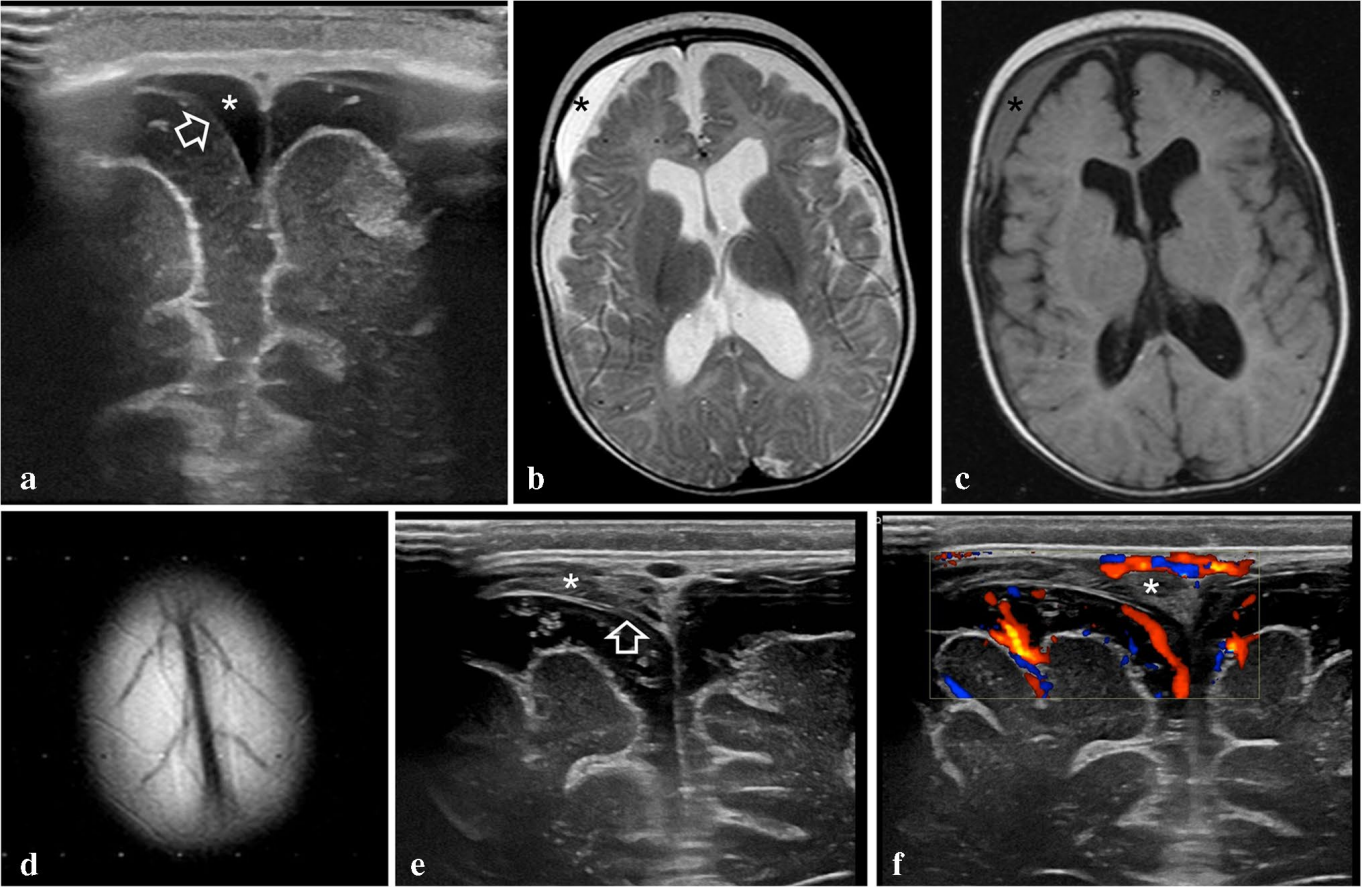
A 3-month-old ex-premature boy with progressive macrocrania and normal development. On ultrasound (US), there was an incidentally discovered subdural collection, confirmed by magnetic resonance imaging (MRI). Fundoscopy, skeletal surveys, social and family history, and clinical follow-up were unremarkable. The diagnosis was subdural collection in the setting of benign enlargement of the subarachnoid spaces. a Coronal US with a high-frequency linear transducer shows enlargement of subarachnoid spaces bilaterally and an anechoic subdural collection (*) displacing the arachnoid dura (*arrow*) on the right. A small linear membrane was also seen on the left. b MRI 2 weeks later. Axial T2-weighted image confirms increased craniocortical width and the presence of a hyperintense subdural collection (*). c MRI, axial FLAIR image, same level as in b. The subdural collection (*) is more conspicuous and restricts the adjacent subarachnoid space. d Axial T2-weighted image at thew convexity shows normal bridging veins. e Repeat ultrasound scan on the same day shows increased echogenicity of the subdural collection (*) while the arachnoid membrane is still visible (*arrow*). f Coronal colour doppler US of the same area confirms lack of vessels within the collection (*)
